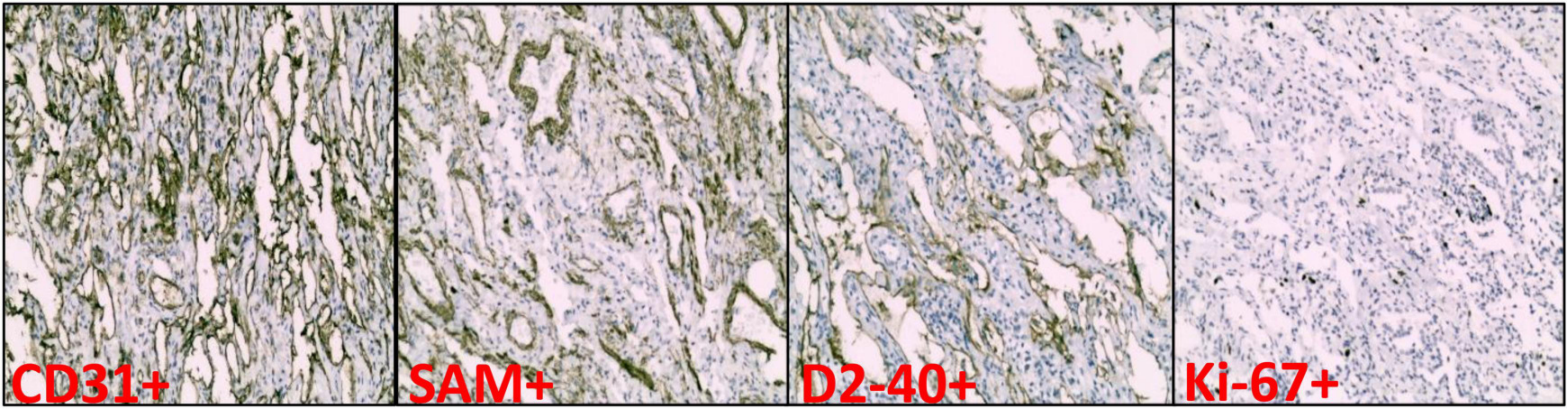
Preoperative chest CT demonstrating interlobular septal thickening, patchy ground-glass shadows, pleural effusion, pericardial effusion, and pericardial thickening.
